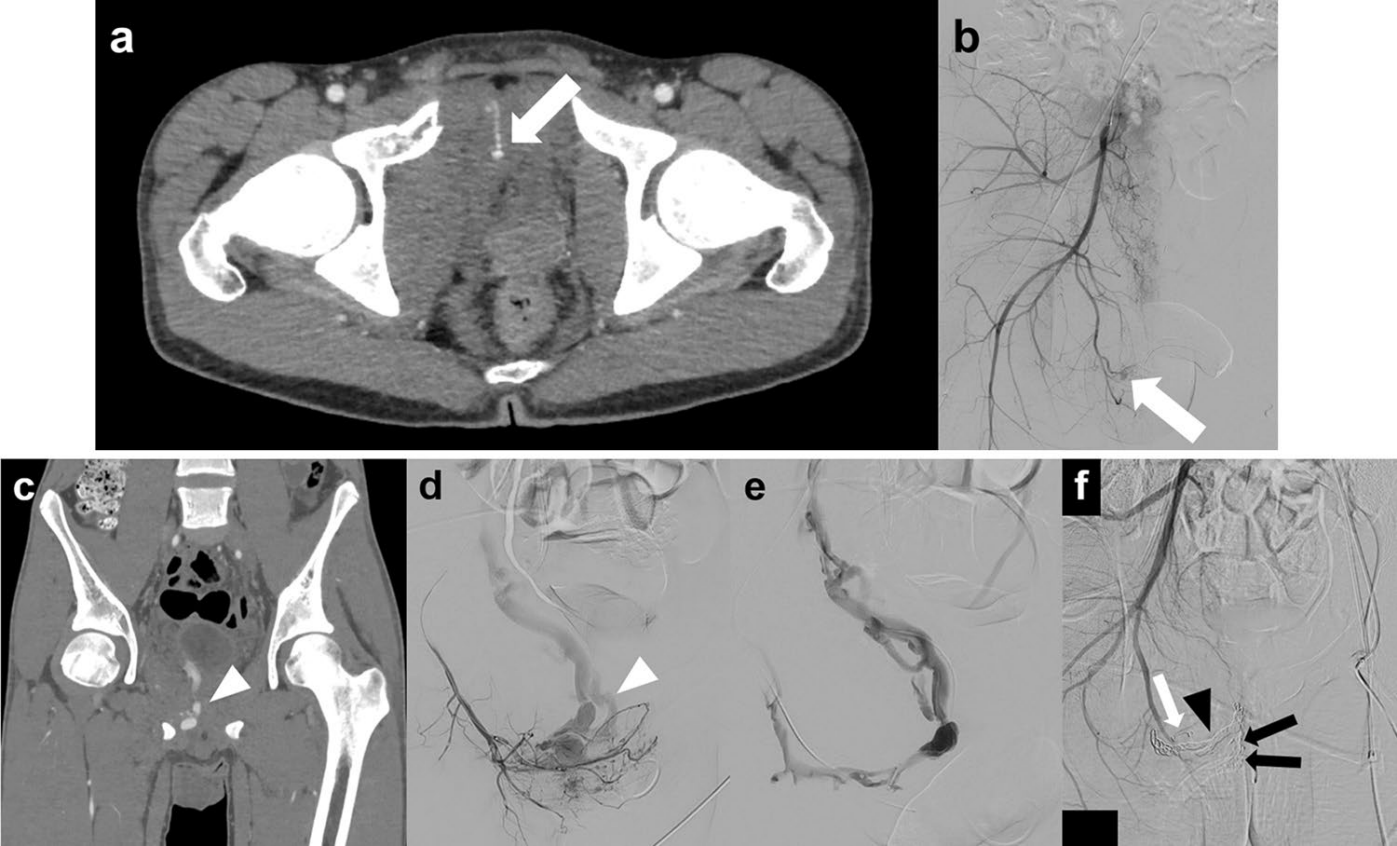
Delayed AVF due to a traffic accident in a 17 year-old boy. a, b CT scan and digital subtraction angiography of the right internal iliac artery obtained immediately after admission show active bleeding (arrows). Non-selective embolization of the right internal iliac artery with gelatin particles has been performed. c, d Coronal view CT scan and digital subtraction angiography of the internal pudendal artery obtained 3 weeks after injury show early filling of a pelvic vein (arrowhead). The AVF has multiple feeding arteries. e A transvenous approach has been used to embolize the drainer vein. f The main drainer vein has been embolized with microcoils (arrowhead), and the feeding arteries have been embolized with NBCA (black arrows), gelatin particles, and microcoils (white arrow). Digital subtraction angiography of the right internal iliac artery shows disappearance of the AVF. *AVF* arteriovenous malformation; *CT* computed tomography
